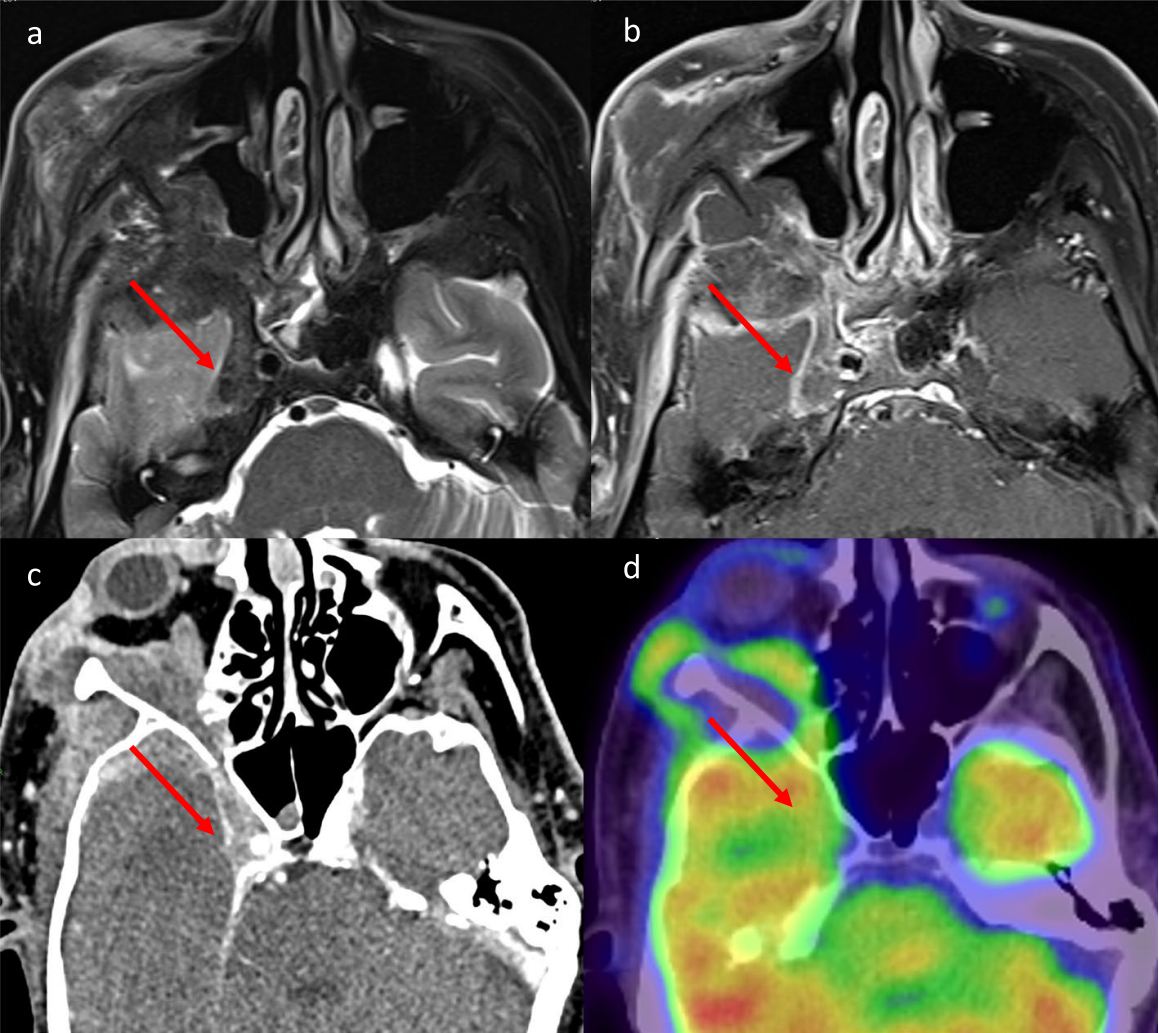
Extensive perineural spread (PNS) not detected by fluorodeoxyglucose-positron emission tomography computer tomography (FDG-PET/CT). A Magnetic resonance imaging (MRI) T2 axial demonstrates extensive right trigeminal nerve PNS in Meckel’s cave. B MRI T1 axial post-contrast fat saturation (FS) demonstrates extensive right trigeminal nerve PNS in Meckel’s cave as a mass with minimal enhancement. C Computer tomography (CT) post-contrast demonstrates mass like extensive right trigeminal nerve PNS in the Meckel's cave. D FDG-PET/CT, extensive right trigeminal nerve PNS not detected on FDG-PET/CT either obscured by FDG avidity in the adjacent brain or minimal FDG avidity in the PNS
